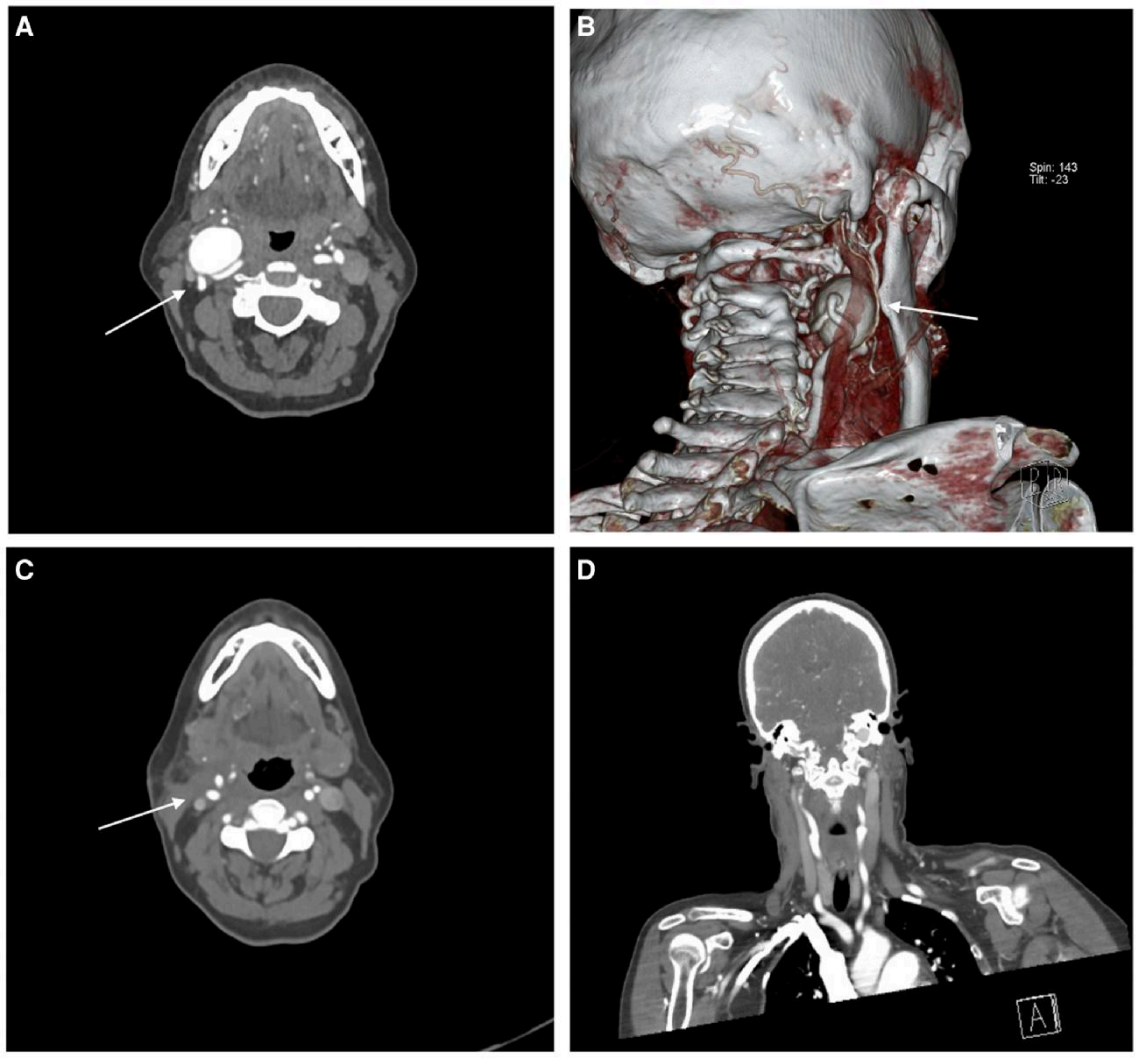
Representative computed tomography images and a three-dimensional reconstruction of the neck and upper cervical region in a patient with an internal carotid aneurysm. (*A*) Axial computed tomography slice demonstrating the oropharyngeal area and adjacent vascular structures, including the right internal carotid aneurysm (arrow). (*B*) 3D volume-rendered reconstruction clearly outlining the internal carotid aneurysm (arrow) and the rest of the arterial anatomy of the neck and skull base. (*C*) Axial computed tomography image obtained after aneurysm repair, showing successful restoration of the arterial lumen and improved vessel contour. (*D*) Coronal view providing a front-to-back overview of the upper cervical region, including the course of the carotid vessels, post-aneurysmal repair.
